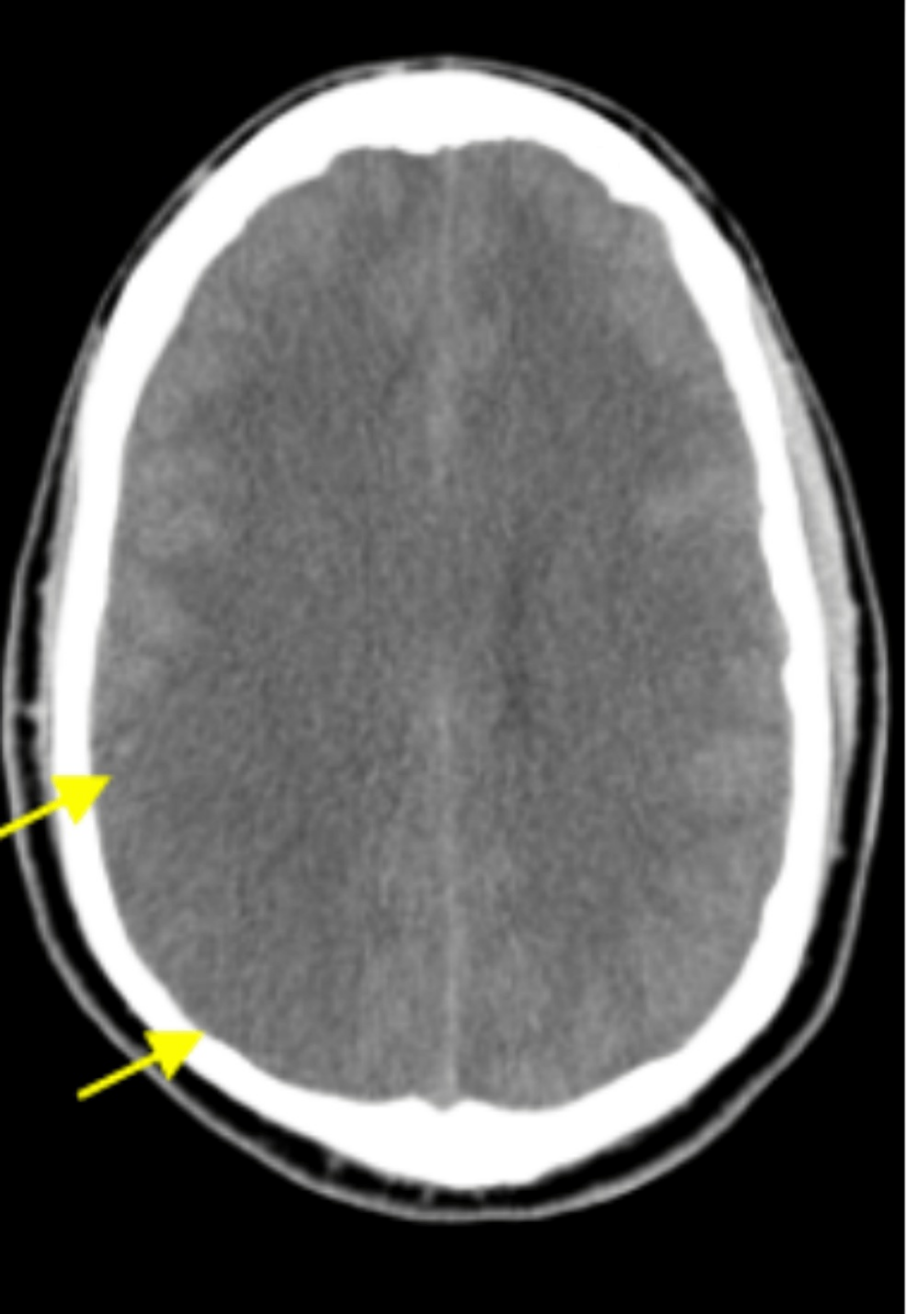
CT brain showing loss of gray white differentiation in parietal lobe suggesting infarct which may be due to PRES
